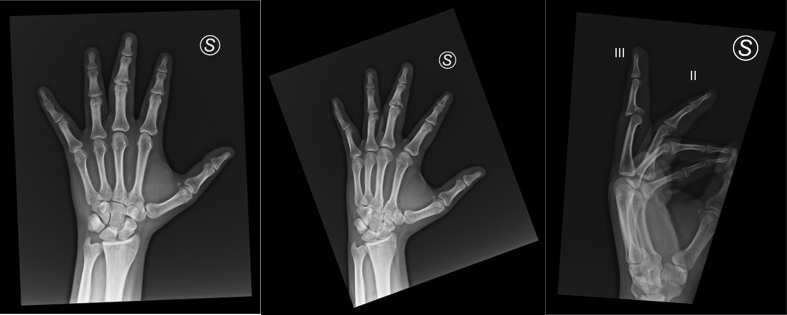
X-ray visualization of PIP joint fracture-dislocation. Lateral X-ray of the left hand displaying a significant fracture-dislocation at the PIP joint of the third digit, labeled as ‘III.’ Notable is the dislocation's alignment compared to the unaffected second digit, labeled as ‘II.’
